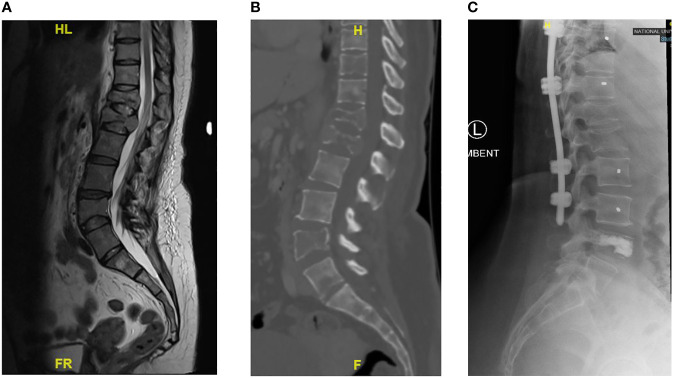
Showing patient with Breast cancer and multiple level vertebral involvement. There were pathological fractures at T12 and L1, SINs score 14 respectively. At T10, the Sins score was 11 and at L4 the Sins Score was 8. Minimally Invasive Posterior Instrumentation was performed from T9 to L3 and vertebroplasty at L4. (A) Pre-operative T2 Sagittal MRI showing multiple levels of vertebral involvement. (B) Pre-operative Lumbar CT scan showing multiple osteolytic lesions. (C) Post-operative Lateral radiograph.
